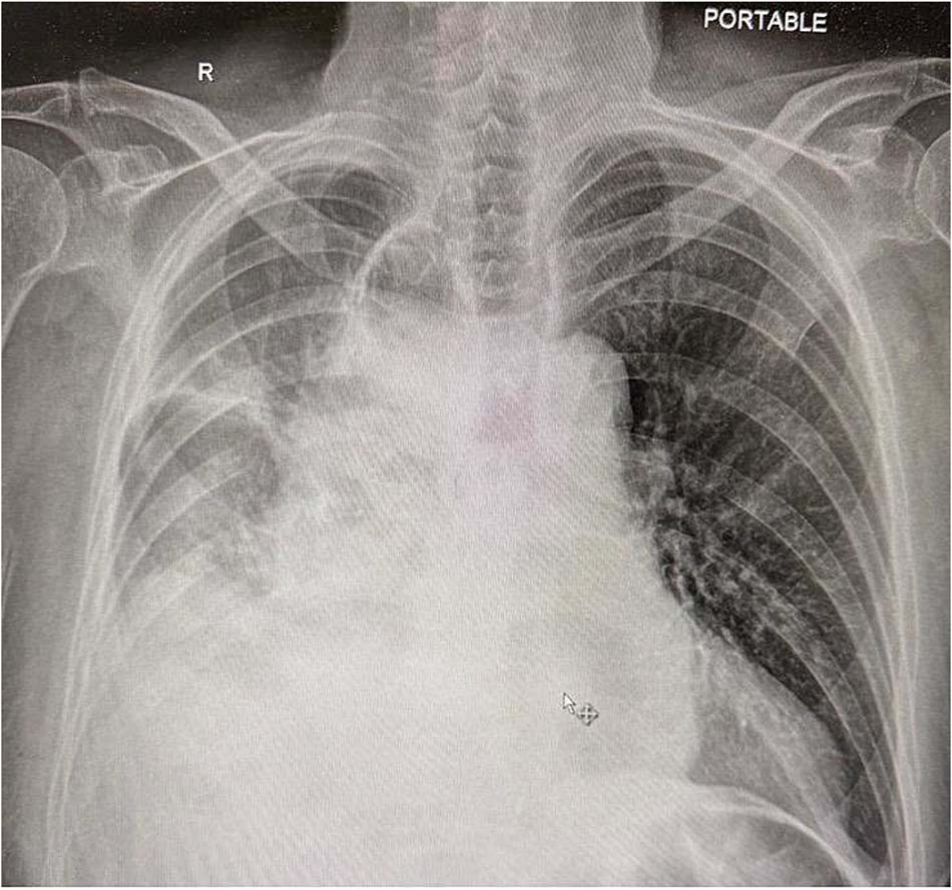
Chest X-ray showing compressed lung and fluid due to herniated small bowel.
